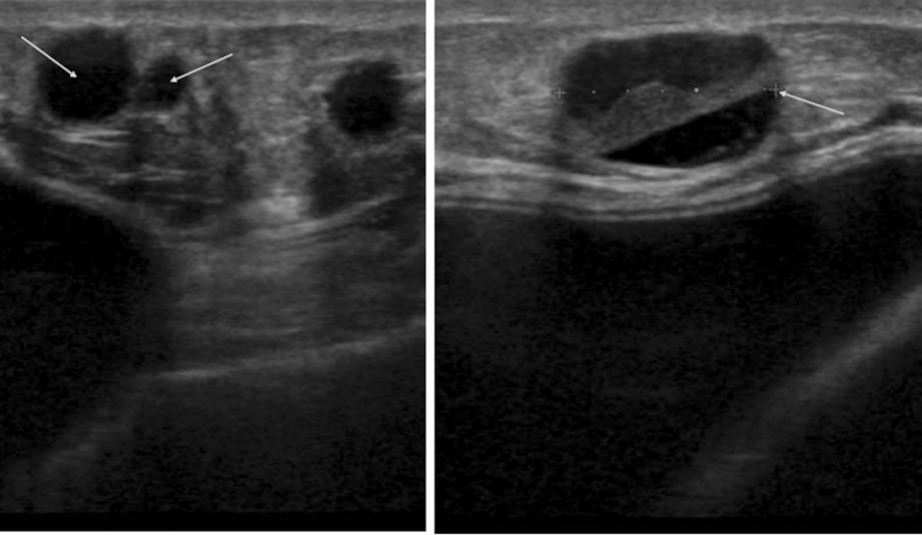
Clinically palpable lump above WLE scar 4 years after grafting (140 ml) in 2008. Mammograms reported as R2. Ultrasound scan showed 14 mm mixed echogenicity lesion reported as likely to be fat necrosis/oil cyst U2, but in view of the solid component ultrasound guided core biopsy performed which confirmed fat necrosis.
